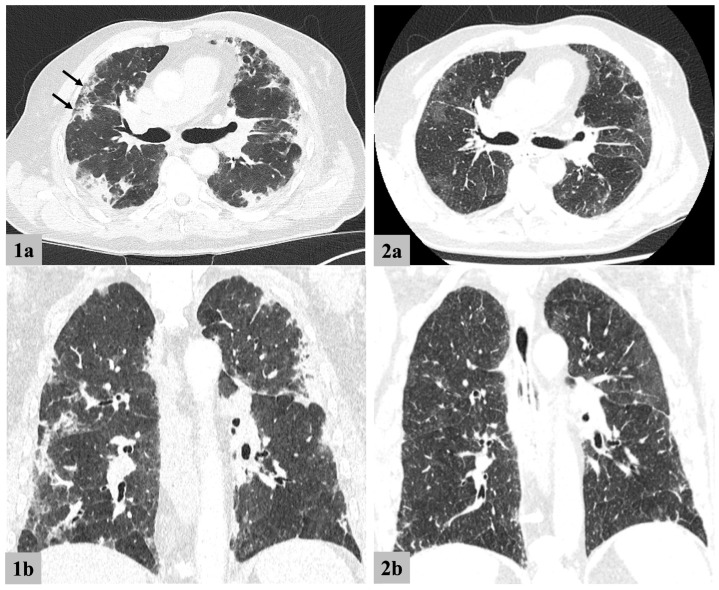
Pulmonary findings on computed tomography (Patient 2). 1 = time of initial diagnosis; 2 = two months later. a = axial reconstructions; b = coronal reconstructions. Marked subpleural consolidations without topographical predominance are seen (marked with arrows). After two months, patchy ground glass opacities remain without notable scarring.
